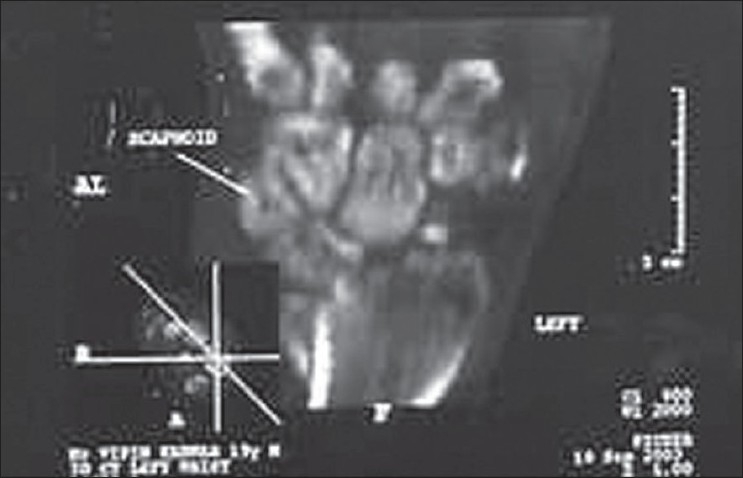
Preoperative CT scan showing nonunion fractured scaphoid with SLAC wrist
